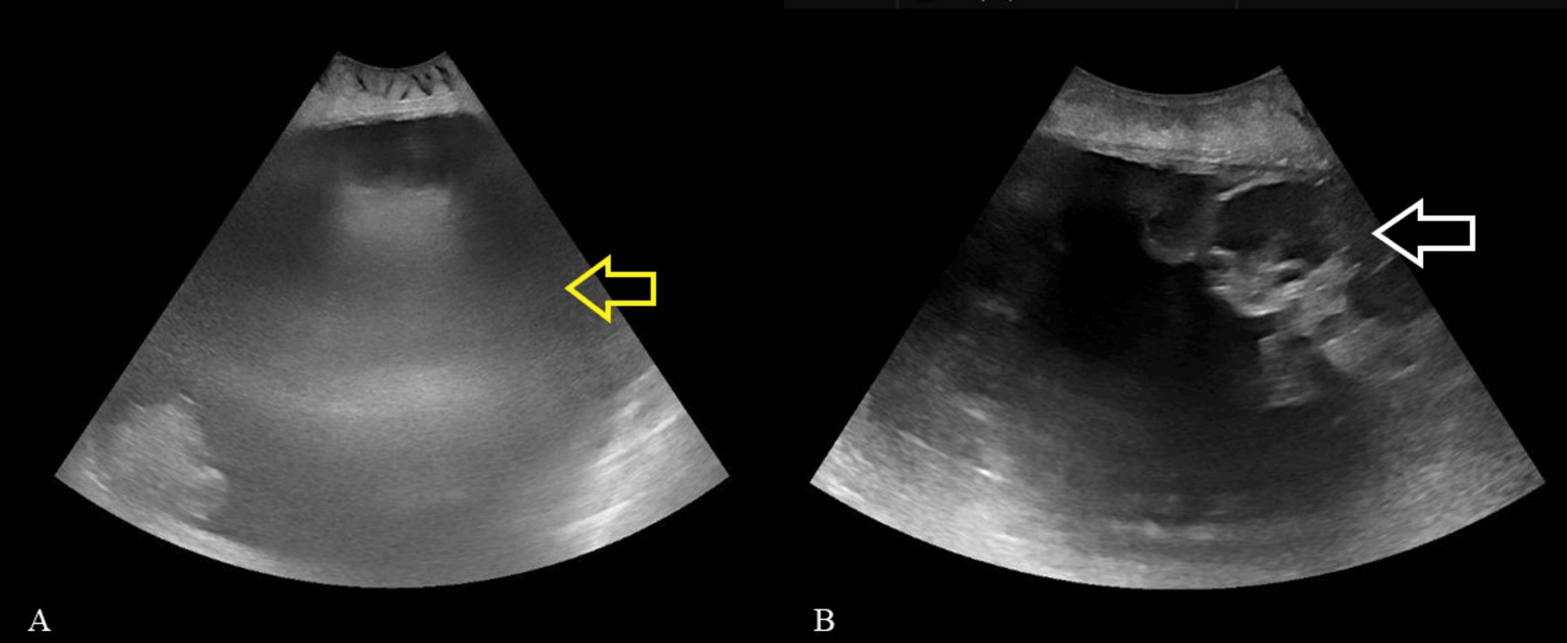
(A) Axial midline transabdominal ultrasound image demonstrating a massive fluid collection occupying nearly the entire peritoneal cavity (yellow arrow). (B) Axial transabdominal ultrasound image at the level of the lower abdomen demonstrating the massive anechoic fluid collection displacing adjacent small bowel loops laterally (white arrow).
